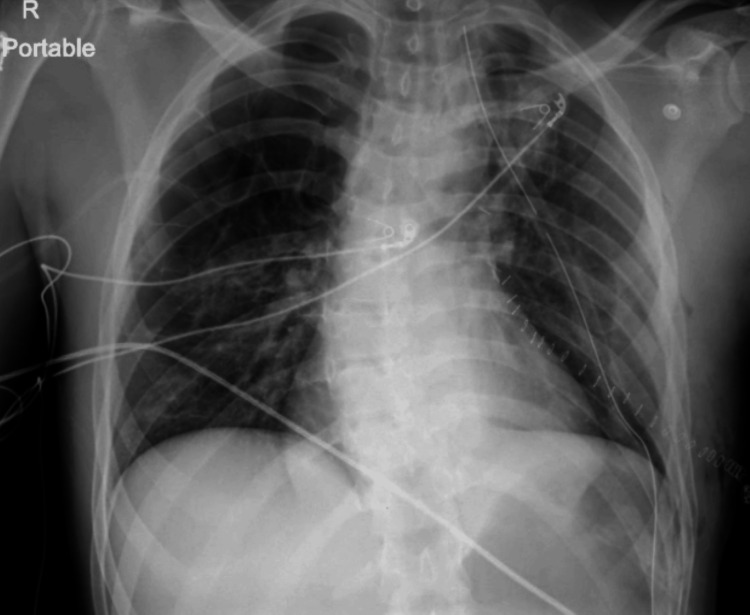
Postoperative chest X-ray with adequate lung expansion
